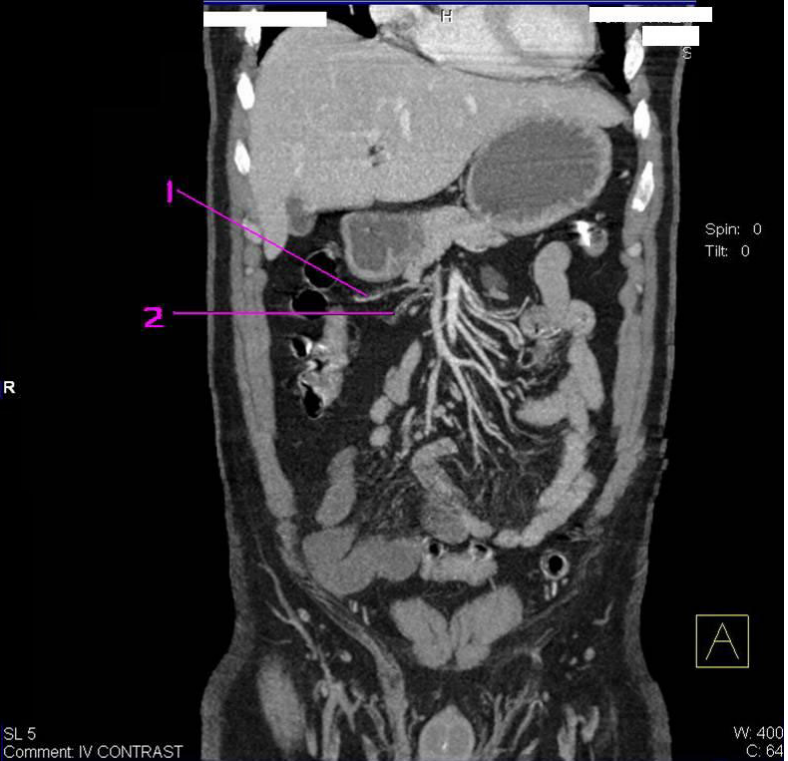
Abdominal Angio CT coronal reconstructed image revealed; no enhancement of the distal IleoColic artery in arterial phase denoting complete thrombosis. Note also; decreased degree of venous enhancement in the IleoColic vein as an effect of arterial thrombosis. (1) IleoColic vein. (2) IleoColic artery.
